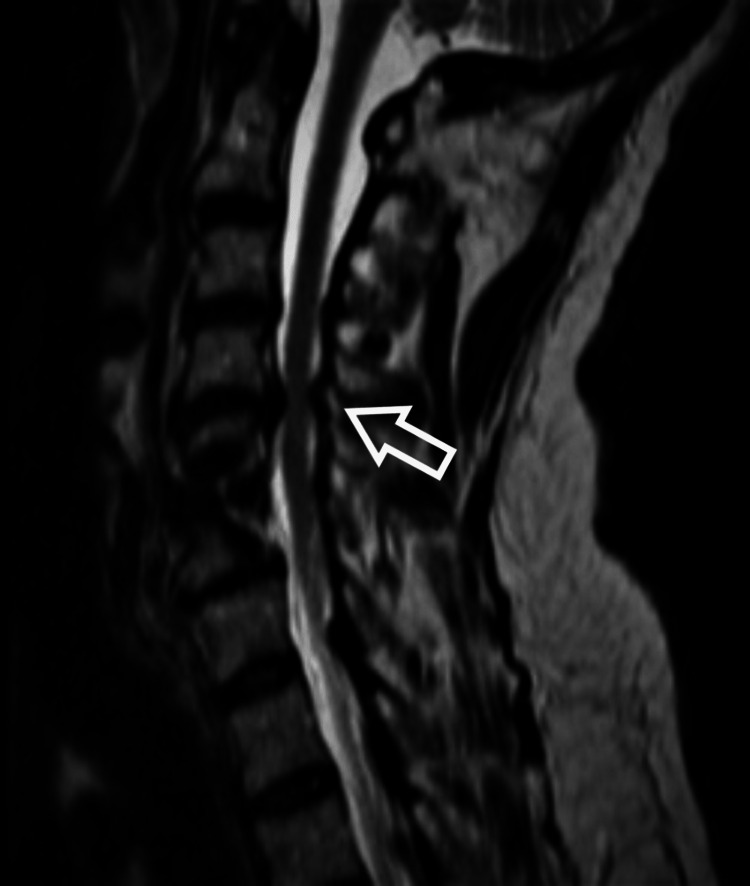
Magnetic resonance imaging scan sagittal view showed stenosis at C4/5. Magnetic resonance imaging scan after six moths showed worsening of cervical stenosis at level above proximal junction level C4/5.
